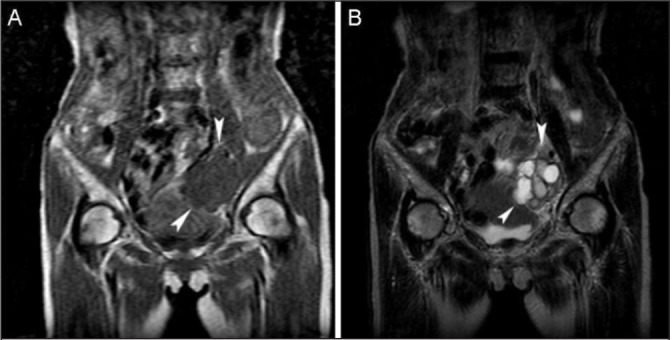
Coronal magnetic resonance imaging (MRI) of the pelvis at presentation: T1 weighted MRI showing an inhomogeneous low intensity mass (A) and T2 weighted MRI showing internal multicystic components with homogeneously high intensities (B)
